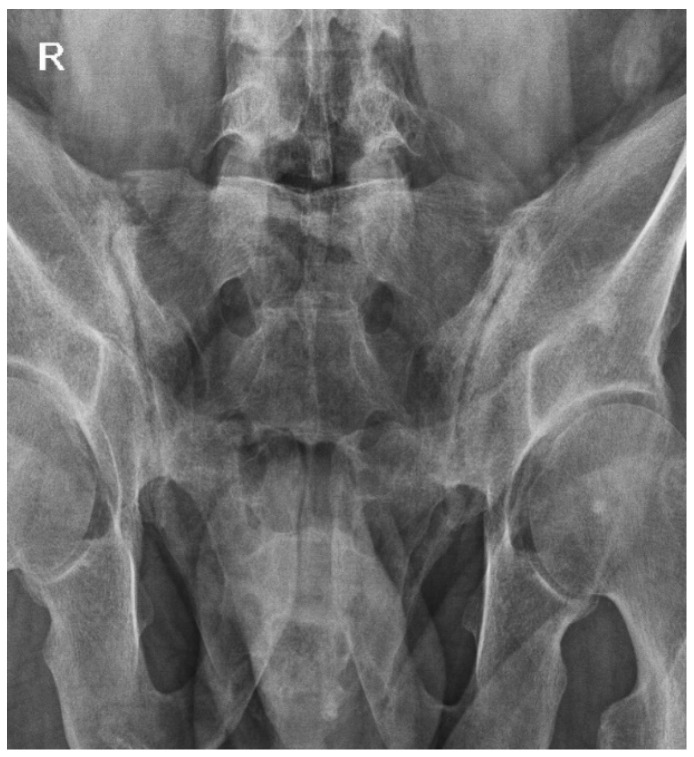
Radiographic imaging of the sacroiliac joints demonstrates bilateral sacroiliitis, classified from stage III to stage IV. Advanced sclerosis is observed bilaterally, accompanied by the presence of superior and inferior ankylosing bridges on the left side, which indicates the progression to advanced ankylosis. On the right side, there are subtle inferior bridging formations, signifying the early stages of ankylotic changes.
